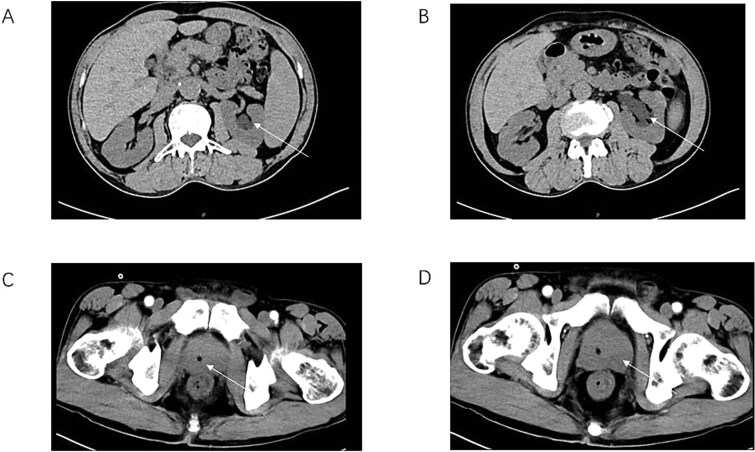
CT of the urinary system (AB) and partial enhancement of the prostate (CD). There was obvious hydronephrosis in the upper renal pelvis of the left ureter in the patient (arrows A&B). The urethra deviates to the right (arrow C), and there is extensive benign prostatic hyperplasia with local mass (arrow D).
